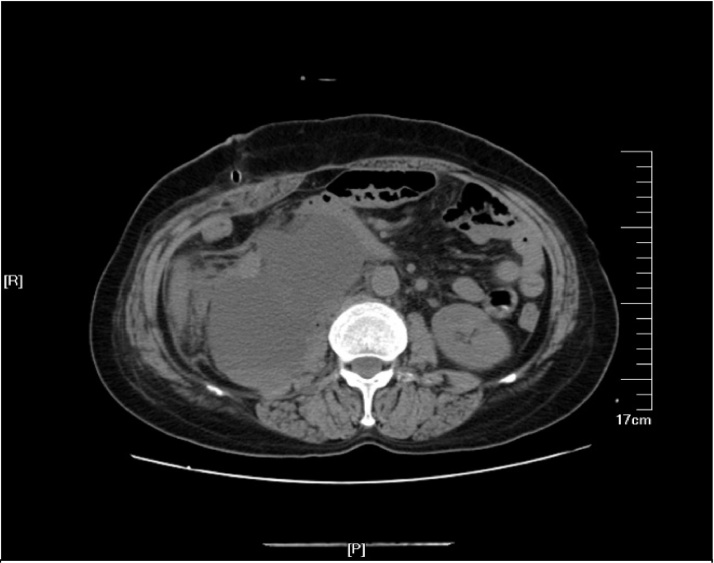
Abdominal CT scan shows a localized retroperitoneal Lymphatic leakage.
